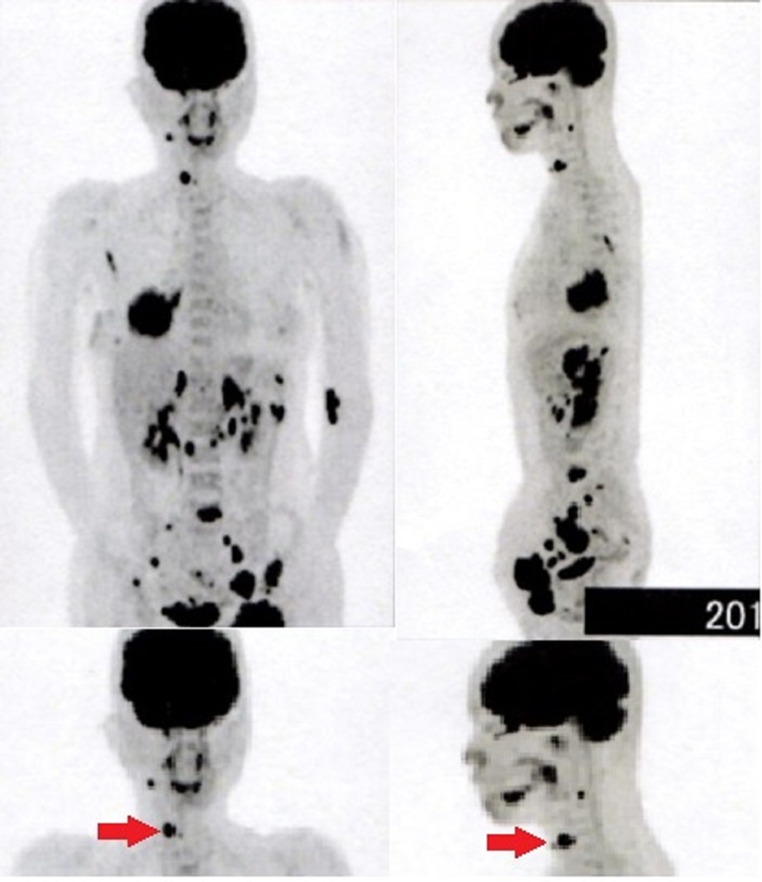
Positron emission tomography shows the highest standardised uptake values to be 14, 17 and 19, respectively, in the right lobe of the thyroid gland, the tumour in the right lower lung lobe and in the left adrenal gland. The right shoulder blade, the left ribs, lumbar vertebrae, the left ilium lymph node, the para-aortic lymph nodes, the bilateral total iliac artery lymph nodes, the left groin and the obturator lymph node also show fluorodeoxyglucose (FDG) accumulation. Arrows show FDG accumulation in the right thyroid lobe.
